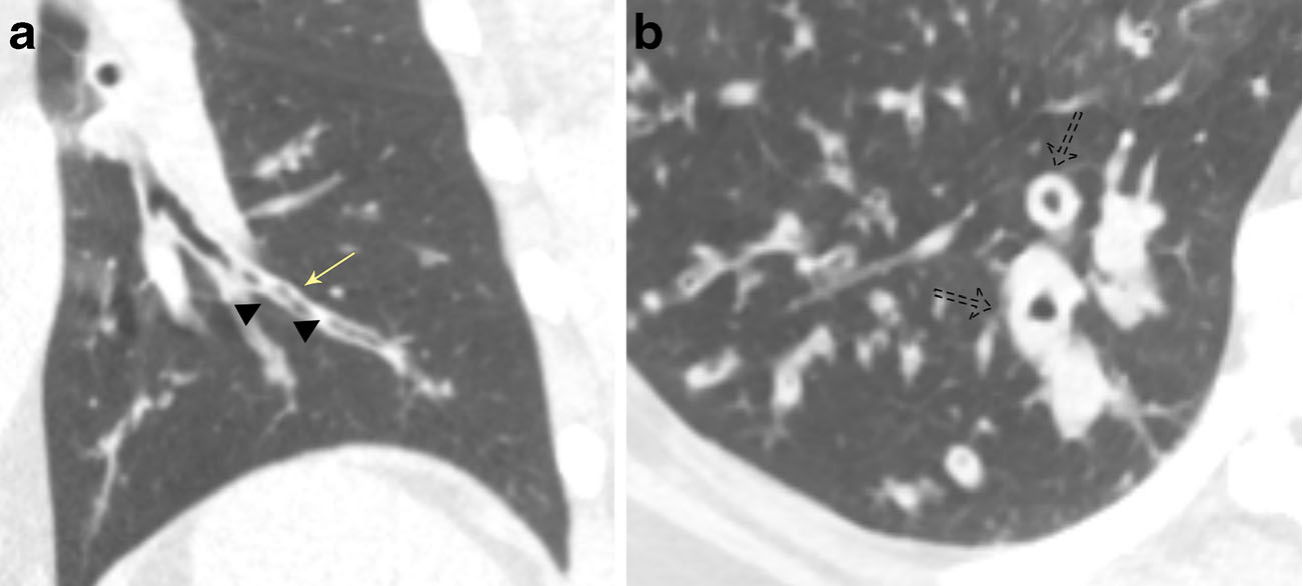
Bronchial wall thickening due to bronchitis. Coronal (a) and axial (b) CT imaging demonstrates bronchial wall thickening and areas of mucous plugging (*arrowhead*). Tram tracking refers to wall thickening in longitudinally oriented bronchi (*solid arrow*) mimicking the parallel rail tracks of a tram whereas peribronchial cuffing (*dashed arrow*) refers to wall thickening of the bronchi seen in cross section, sometimes referred to as the “donut sign”
